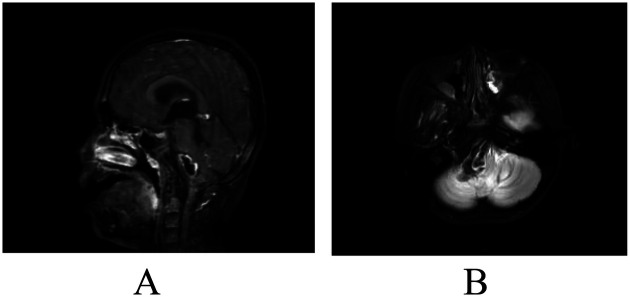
(A,B) MRI demonstrates a fusiform dilatation of the right vertebral artery (V4 segment) measuring approximately 14 × 14 × 31 mm, with compression and leftward displacement of the adjacent medulla and pons.
